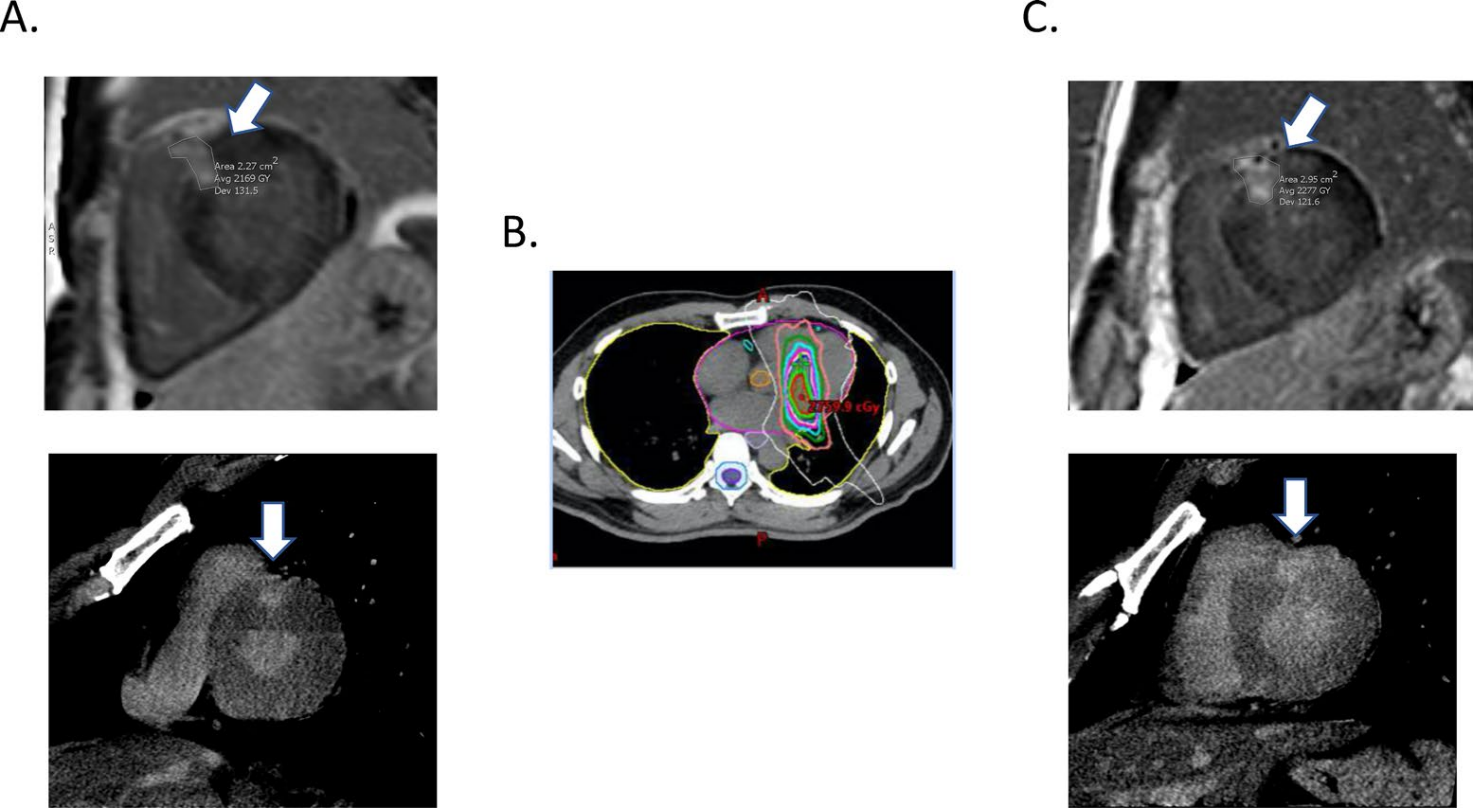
Pre- and post- treatment imaging comparison. (A) Pre-treatment CMR (upper part) and dual-energy CT (lower part) of patient 3 showed delayed enhancement of basal antero-septum (white arrow). (B) SBRT contour of patient 3. (C) Post-treatment CMR (upper part) and dual-energy CT (lower part) of patient 3 showed mildly increased delayed enhancement of basal antero-septum (white arrow). Pre-treatment scar area was 2.27 cm^2^, and post-treatment scar area was 2.95 cm^2^. The radioablation dose distribution figure was created by using treatment planning system (version 15.6, Eclipse, Varian Medical Systems, Palo Alto, URL: http://www.MyVarian.com).
